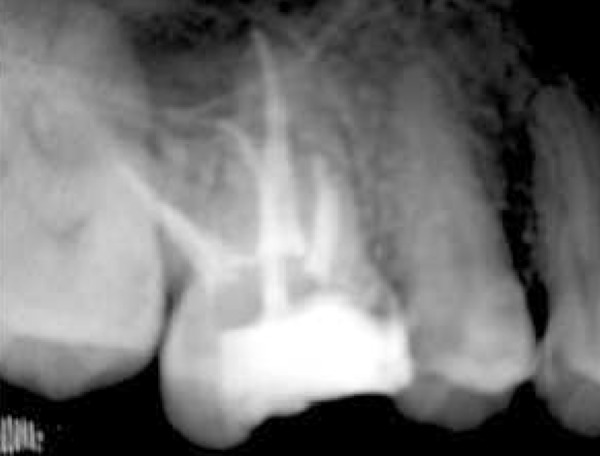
The final radiograph of tooth #16
